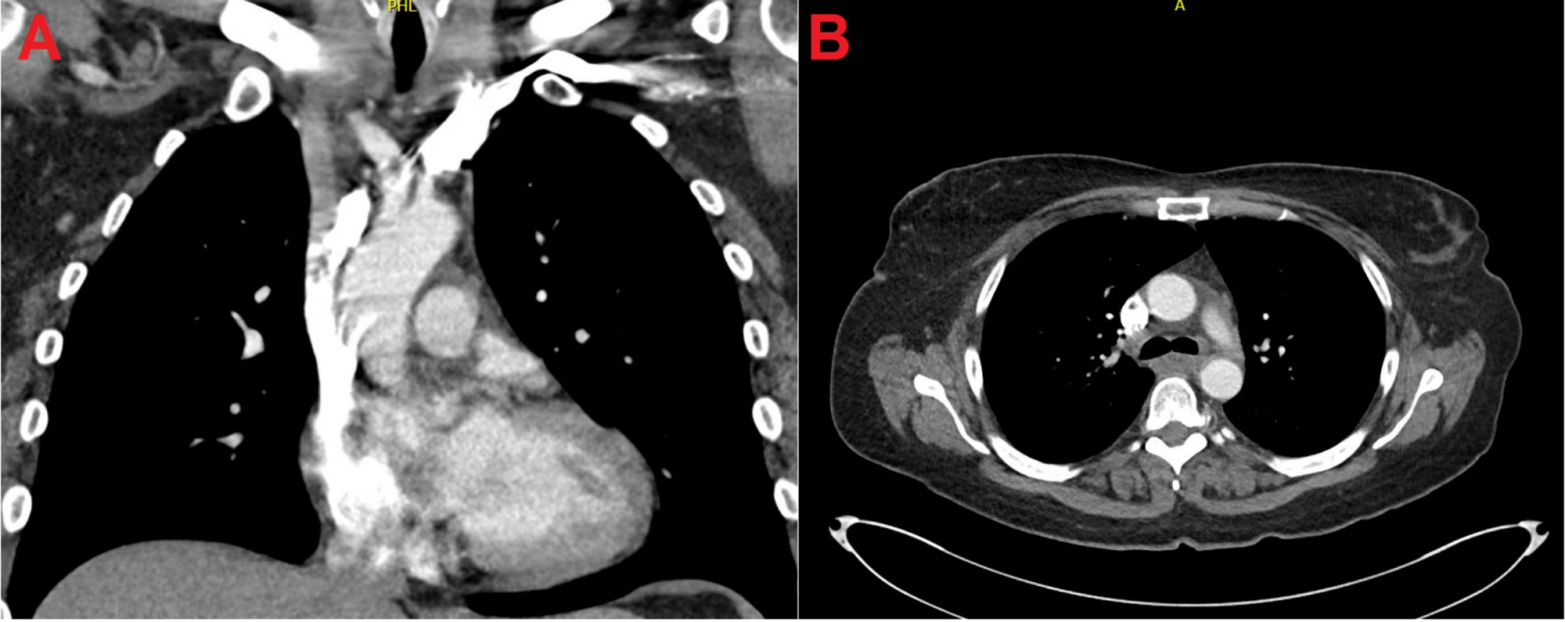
Computed tomography of the chest with intravenous contrast after treatment Coronal (A) and axial (B) section of the chest showing resolution of the mediastinal mass after chemoradiotherapy
